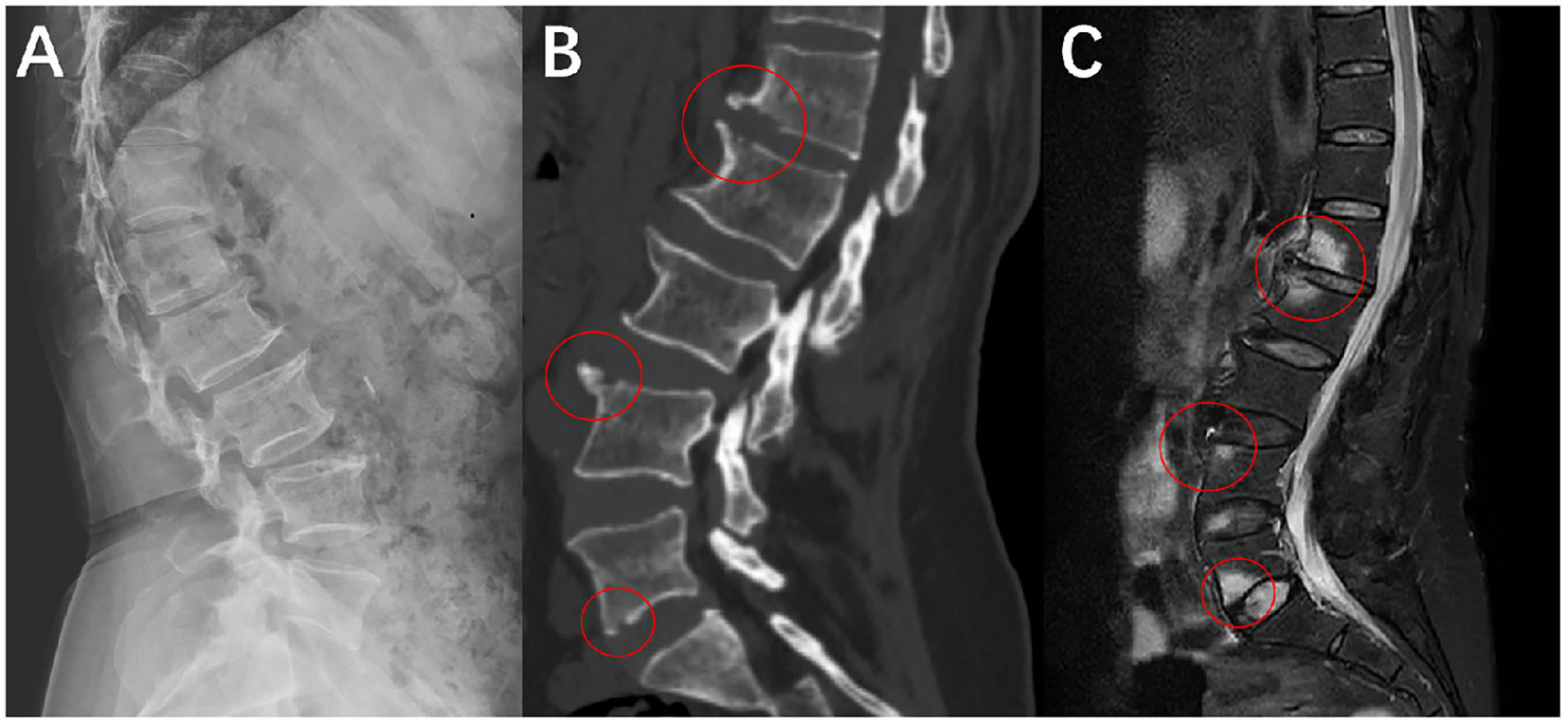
The image shows a mixed change of osteogenesis and bone destruction. (A) X-ray examination, shows bone hyperplasia at the anterior edge of the vertebral body in the lateral view of the lumbar spine. (B) CT examination, and the position within the red circle can more clearly demonstrate the coexistence of bone hyperplasia and bone destruction. (C) MRI examination, and the red circle indicates vertebral edema and local bone marrow reaction.
